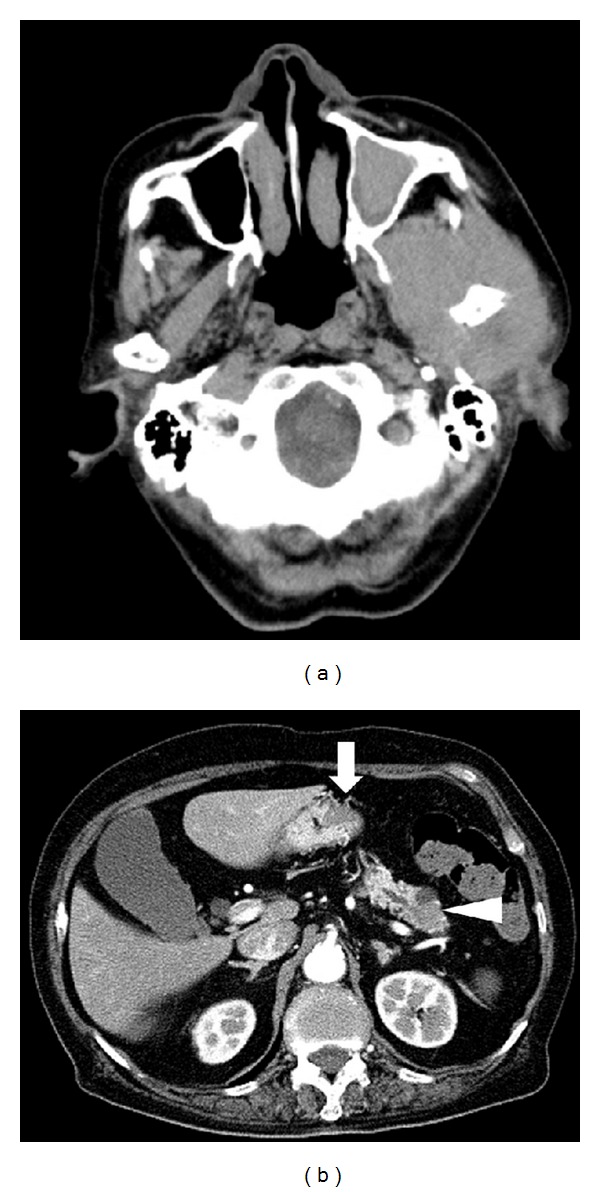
CT scanning images. A soft tissue density lesion 6 cm in diameter in the left temporomandibular joint is shown (a). Multiple hypovascular tumors were also detected in the stomach (arrow) and the pancreas (arrowhead) (b).
